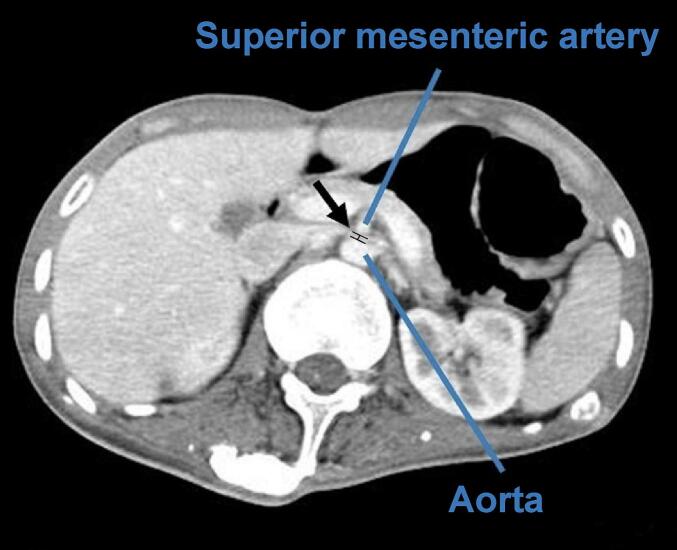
Abdominal CT showing extrinsic compression of the third part of the duodenum between the aorta and superior mesenteric artery. Arrow: Aortomesenteric distance measuring at 4.3 mm (normal value, >10 mm).
